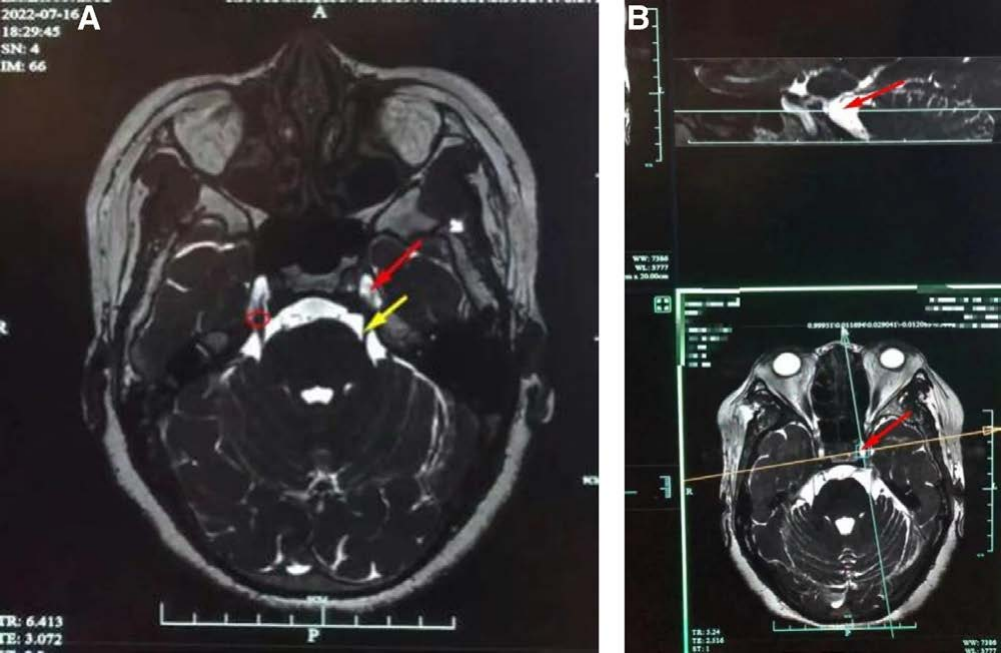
MRI scans of the left trigeminal nerve. Red arrow: the trigeminal ganglion; Yellow arrow: the trigeminal nerve; Red circle: the trigeminal nerve pore.
